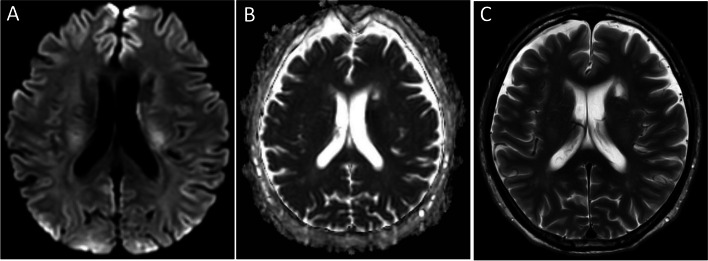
Magnetic resonance imaging brain. A Diffusion-weighted imaging (DWI). B Apparent diffusion co-efficient (ADC). C T2 weighted (T2W). Restricted diffusion in left corona radiata periventricular region on DWI and ADC with no associated T2W hyperintensity, suggestive of an acute infarct
